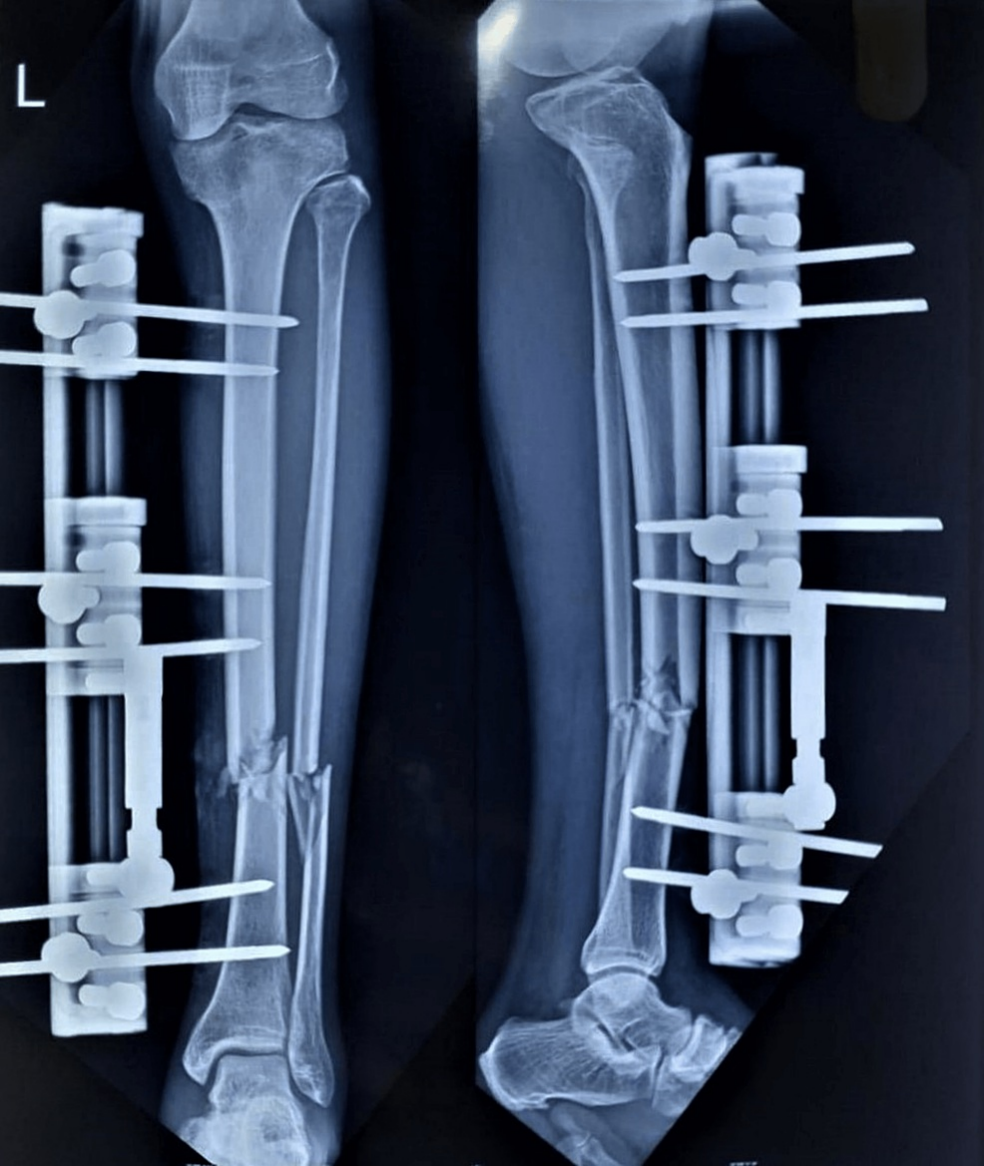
Six weeks postoperative X-ray (anteroposterior and lateral view)
